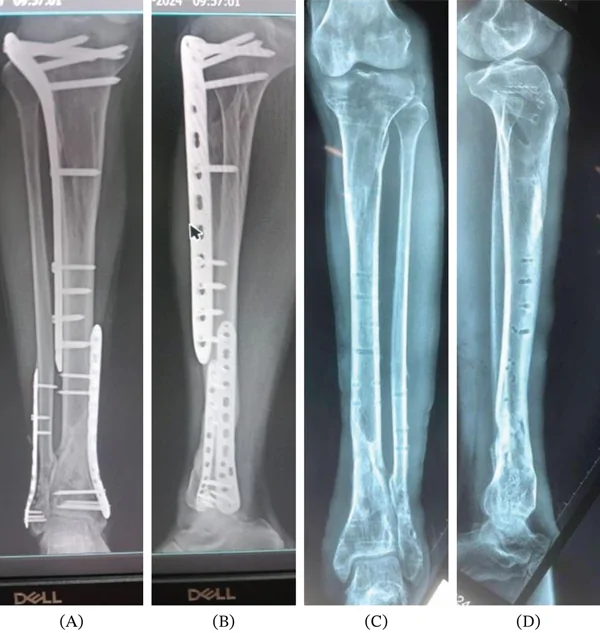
Eighteen‐month radiographs before and after elective implant removal. (A–B) Anteroposterior and lateral views before removal demonstrate maintained alignment and complete union. (C–D) Corresponding views after implant removal demonstrate preserved alignment and healed fracture sites. No image enhancement other than routine sizing and panel labelling was applied for this revision.
